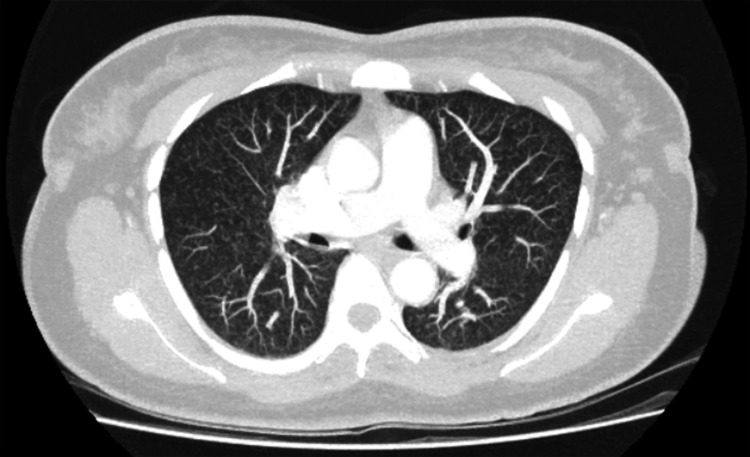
CT of the chest showing extensive nodularity throughout both lungs, suggestive of miliary tuberculosis.
